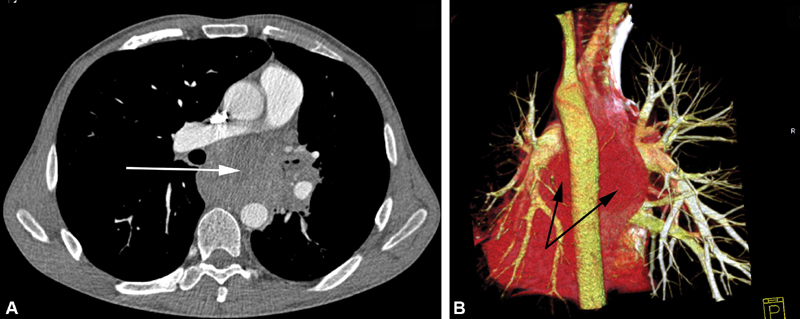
Computed tomography (CT) scan (
